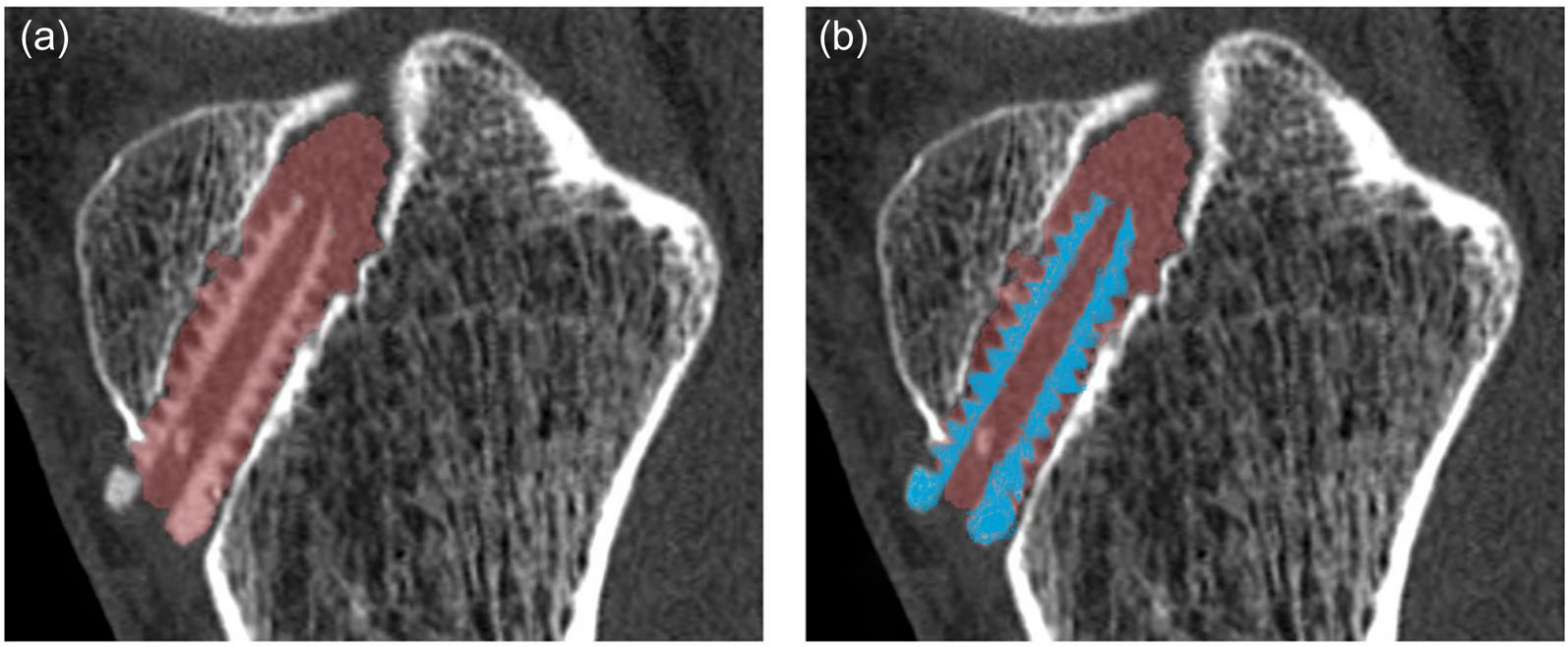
Examples of identification of the automated tunnel area (a) and screw area (b) in single sagittal reconstructed computed tomography sections.
